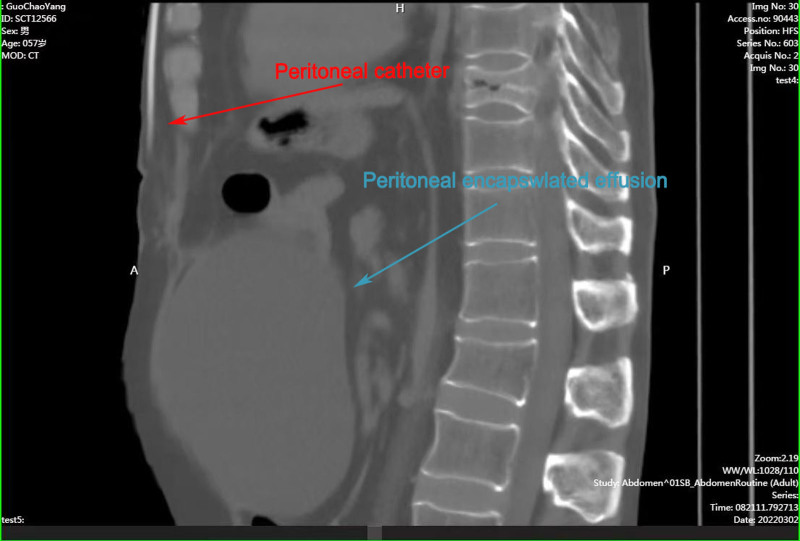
Abdominal CT showed the peritoneal catheter and peritoneal encapsulated effusion. The red arrow indicates the peritoneal catheter, while the blue arrow points to the peritoneal encapsulated effusion. CT = computed tomography.
